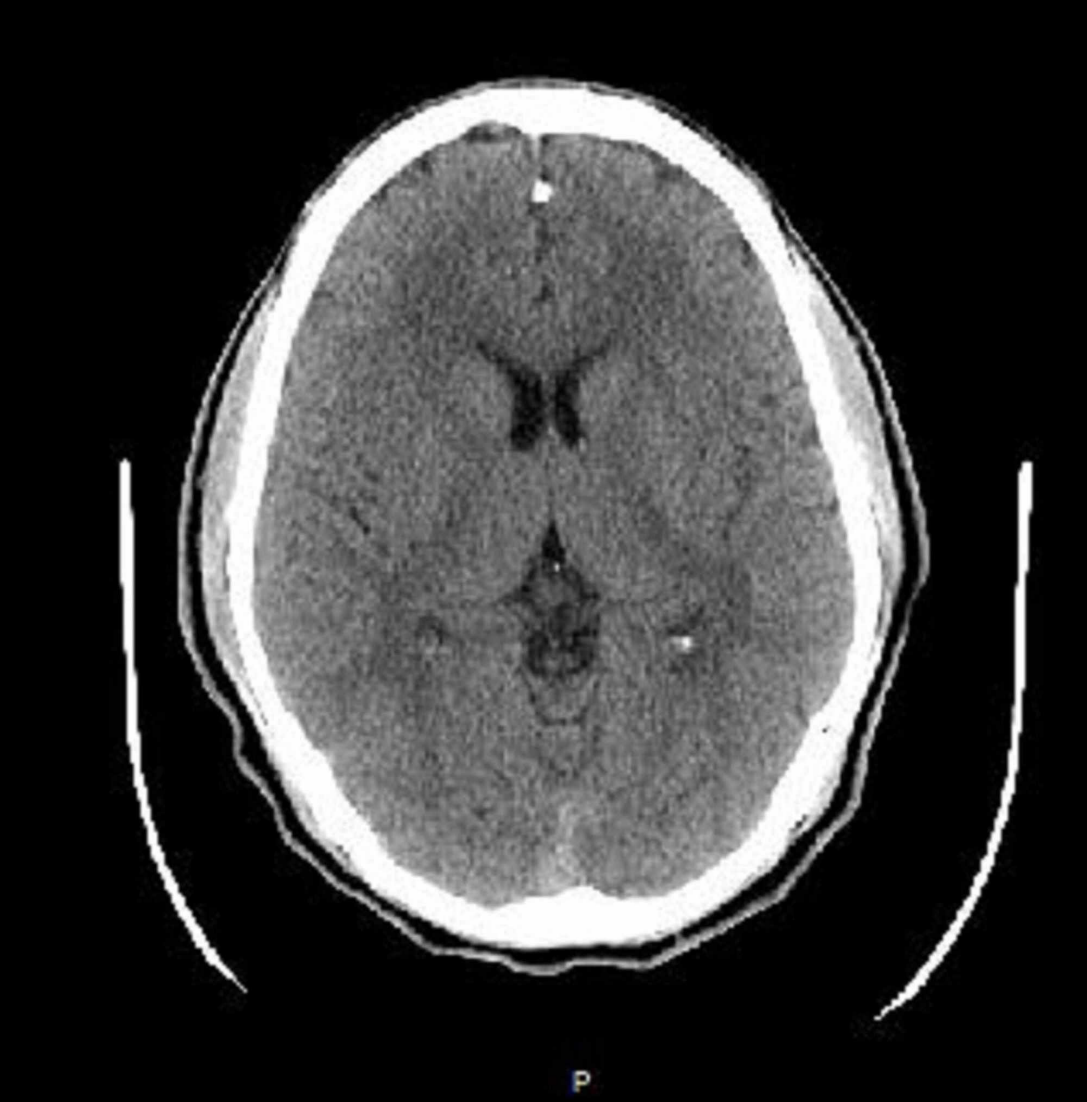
Head CT scan without contrast on admission showing no abnormal features.
